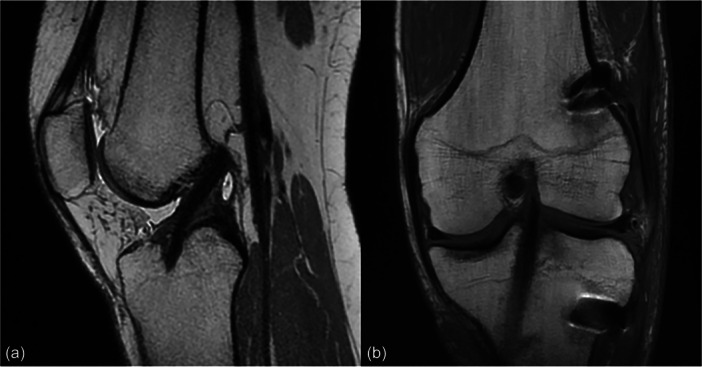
Postoperative MRI scan of a patient following over‐the‐top ACL reconstruction in sagittal view (a) and coronal view (b). ACL, anterior cruciate ligament; MRI, magnetic resonance imaging.
